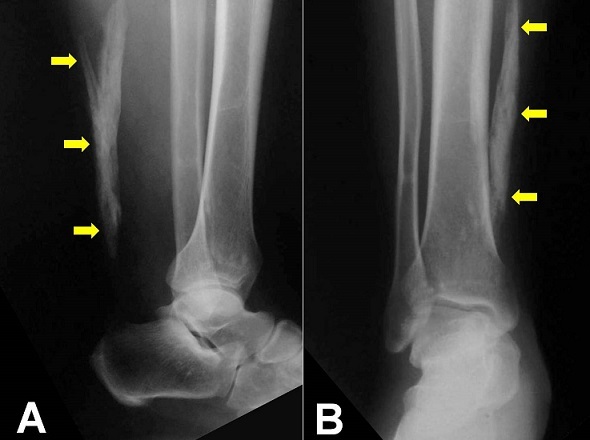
Plain radiographs of the right leg and ankle. Lateral view (A) and antero-posterior (B) view revealing a 10 centimeters ossification within the right Achilles tendon without fracture
